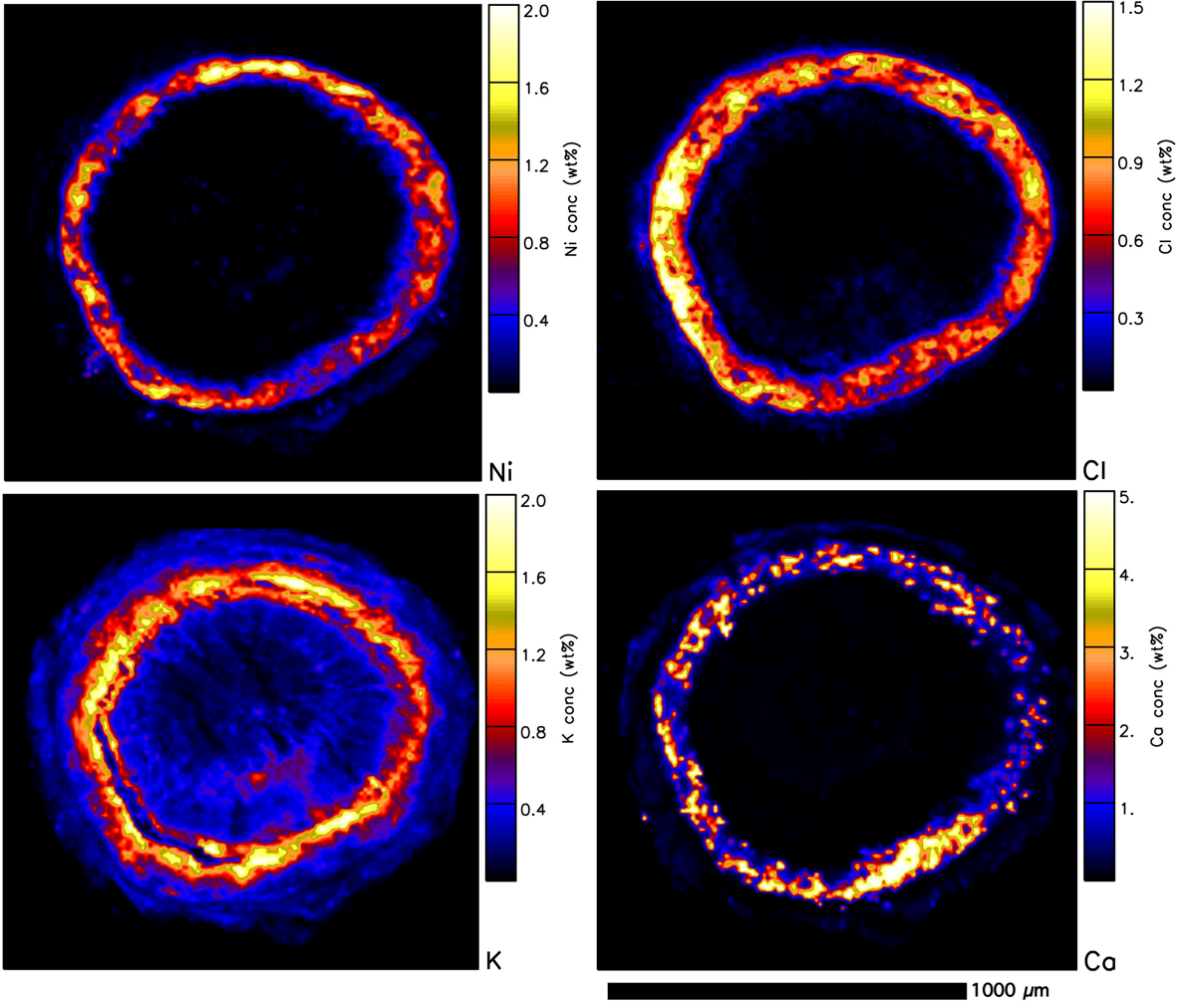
Individual elemental particle-induced X-ray emission (PIXE) maps of a freeze-dried *P. rufuschaneyi* root section showing Ni, Cl, K, and Ca maps. Acquired at the nuclear microprobe facility of iThemba LABS.
